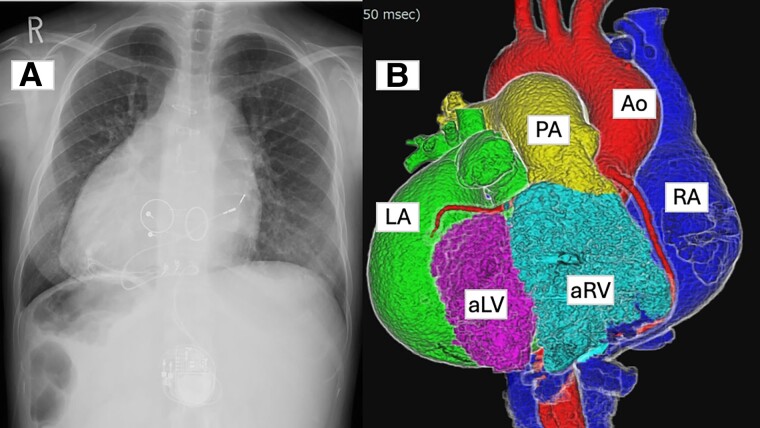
Chest radiography (*A*) and cardiac computed tomography (*B*) showing dextrocardia and incomplete atrioventricular septal defect. Ao, aorta; aLV, anatomical left ventricular; aRV, anatomical right ventricular; LA, left atrium; PA, pulmonary artery; RA, right atrium.
